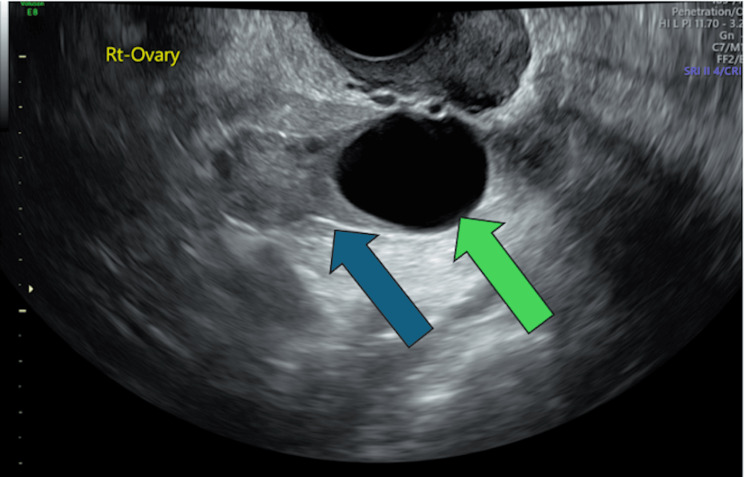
Transvaginal ultrasound: The right ovary (blue arrow) is seen, along with the dominant follicle (green arrow).
